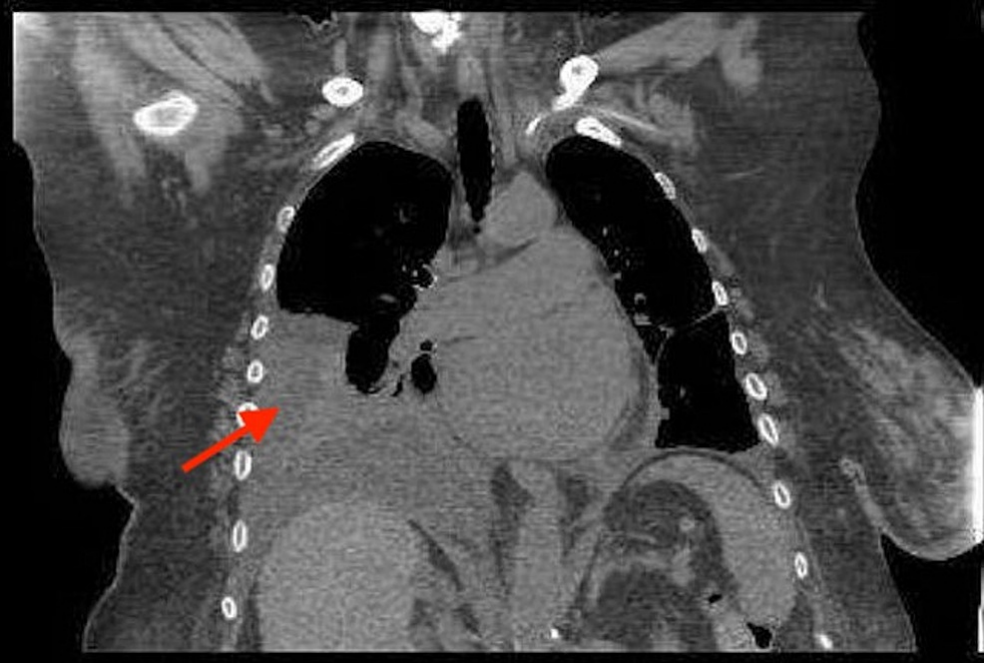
CT chest without contrast coronal view showing moderate right pleural effusion (red arrow).
